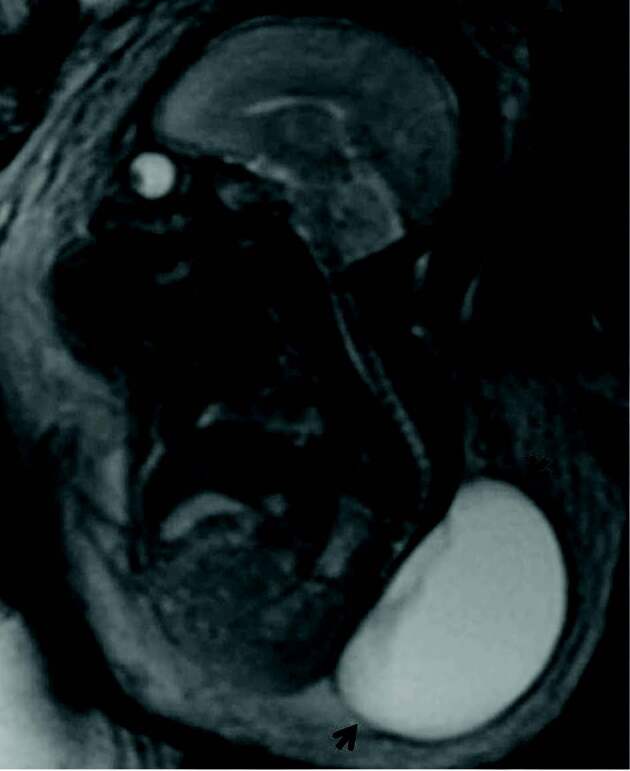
Sagittal T2 SSFSE fetal MR image reveals large lumbosacral myelomeningocele sac (arrows).
